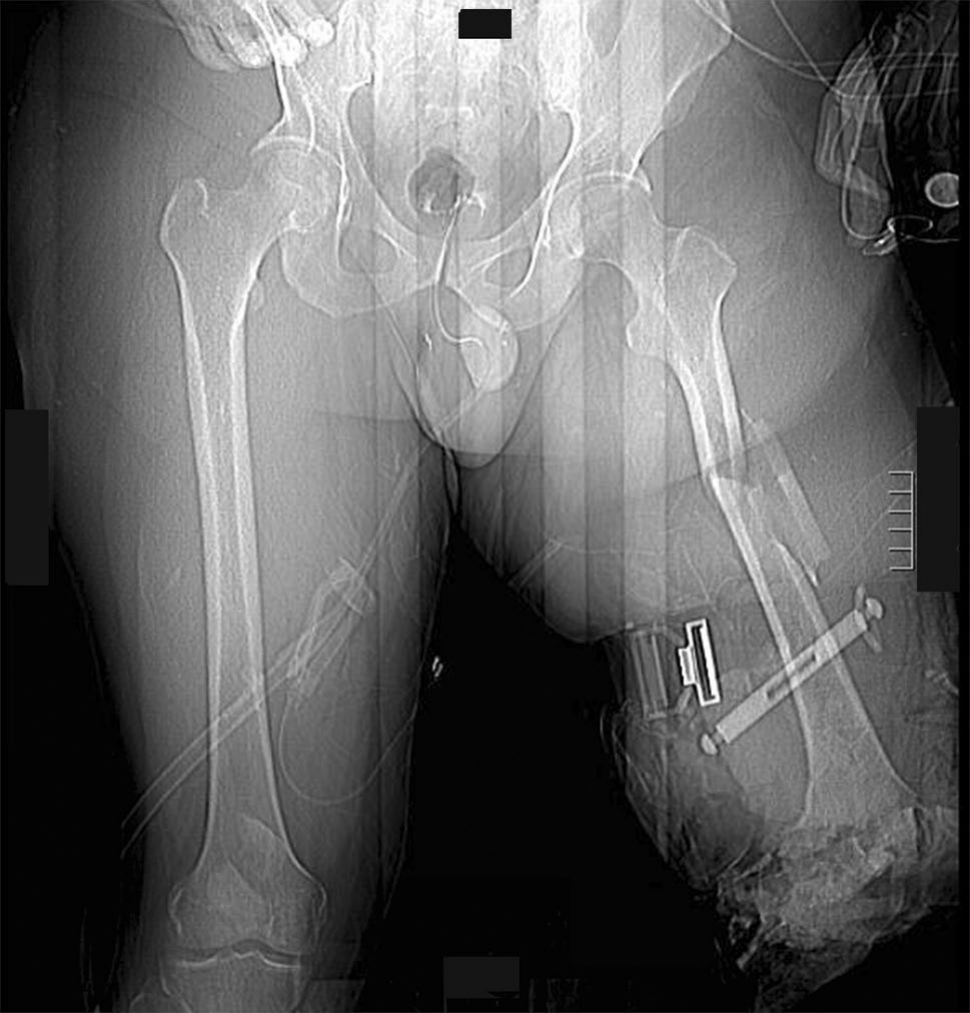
Anterior–posterior scout image from computed tomography study obtained on night of admission showing left comminuted femoral shaft fracture, circumferential tourniquet around leg and traumatic through-knee amputation
